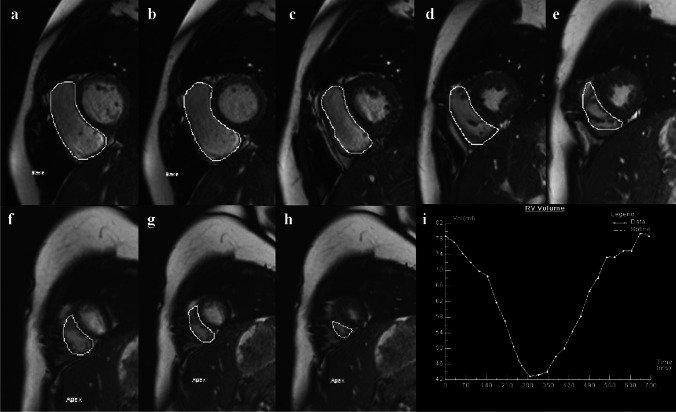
Partial right ventricular (RV) short-axis views obtained by cardiac magnetic resonance (MR) in a 10-year-old boy with patent foramen ovale, depicting RV basal segments (a, b), mid-cavity segments (c–e), and apical segments (f–h). i Volume-time curve of the RV generated by cardiac MR image analysis software
